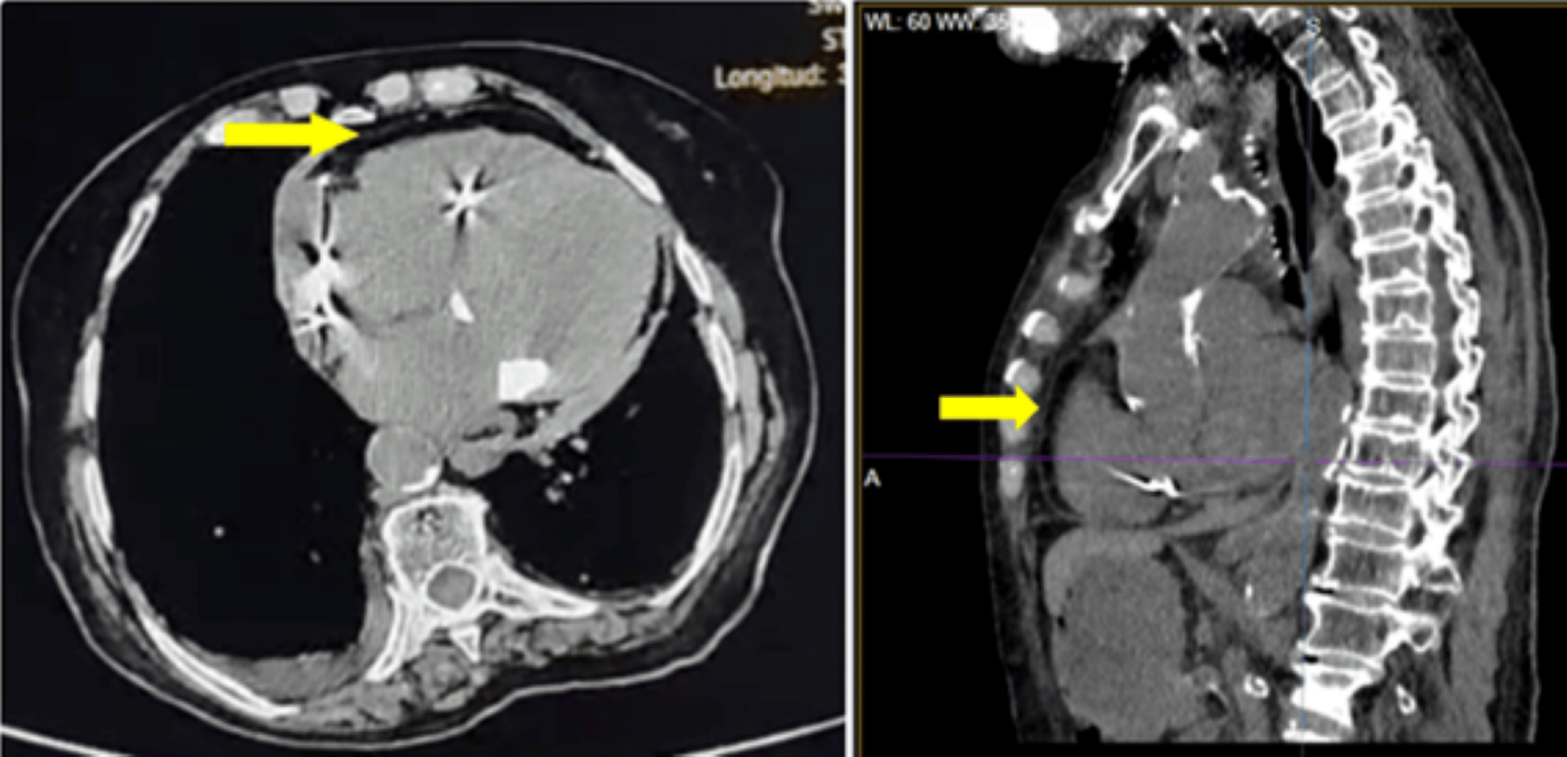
Non-contrast Chest Computed Tomography Axial (left) and sagittal (right) computed tomography images demonstrate a high-attenuation pericardial effusion consistent with mild hemopericardium (arrow) and focal mural heterogeneity along the anterior right atrial wall (arrow), suggestive of a contained microhematoma at the lead fixation site. Additional findings include a small-to-moderate right pleural effusion, diffuse coronary and aortic calcifications, and subtle basal interstitial thickening consistent with mild interstitial lung disease.
